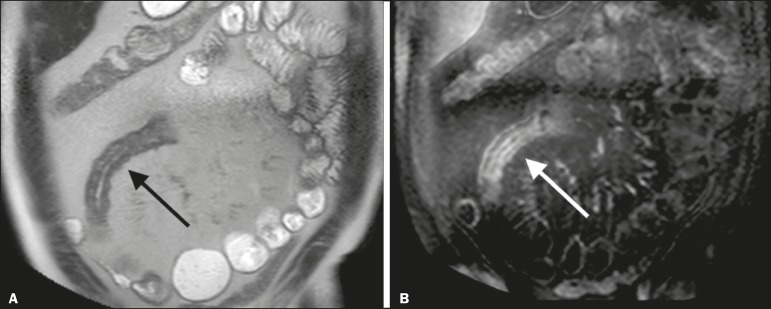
Coronal T2-weighted MRE sequence (A) and coronal, paramagnetic contrast-enhanced, fat saturated T1-weighted MRE sequence (B), showing impairment of the long segment of the distal ileum with stratification of the layers (arrows). Note also the fat proliferation adjacent to that segment, separating it from the other ileal loops.
